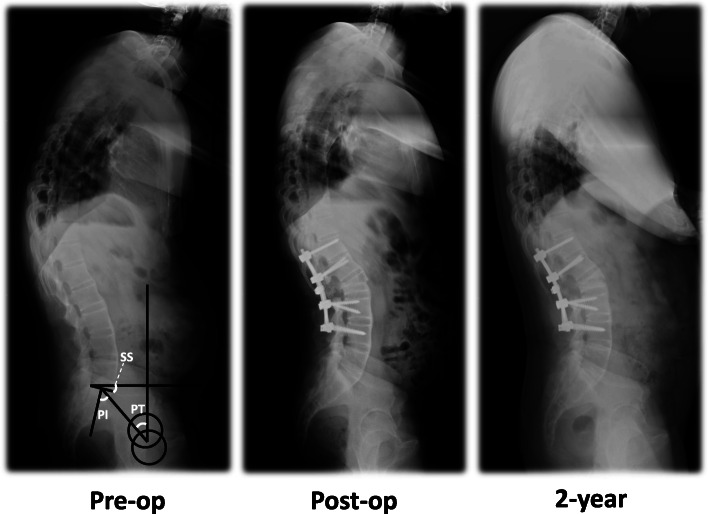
Preoperative, immediate postoperative and 2-year postoperative standing anteroposterior and lateral digital radiographs were reviewed
